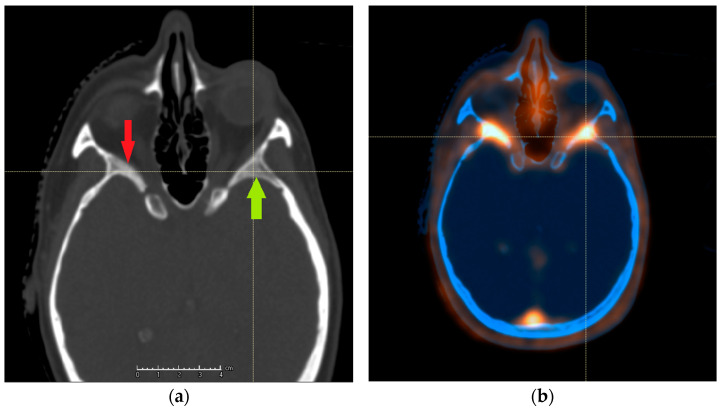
Example of an intraosseous extension of meningioma not properly recognized on CT: (a) the right side displays suspicious hyperostosis (red arrow), and the left side appears to have the normal bone structure preserved (green arrow). PET/CT revealed meningioma extension on both sides (b).
